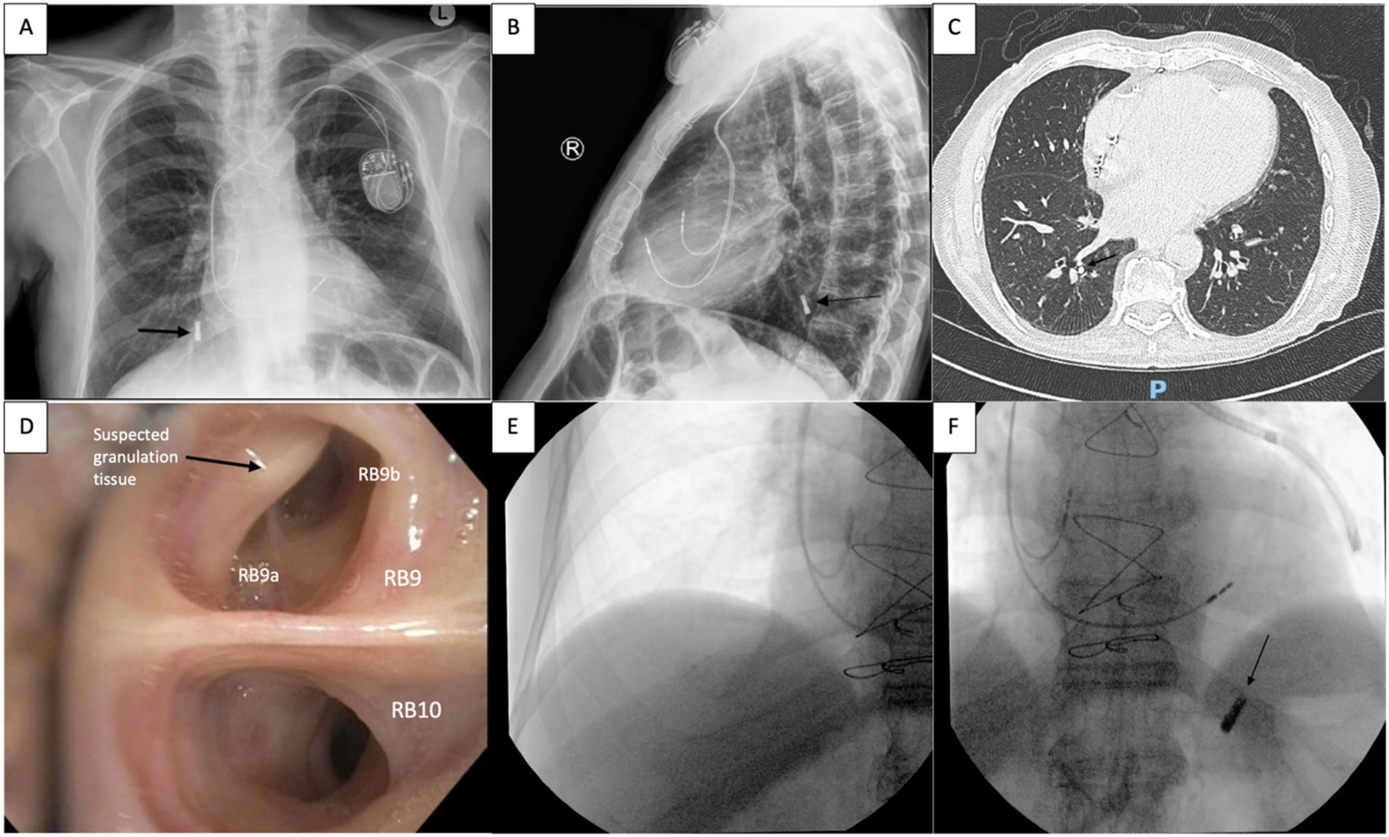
Respiratory phase: (A, B) Chest X‐ray showing metallic foreign body in the right lower lobe → (C) CT Chest confirming foreign body within distal posterior basal segment of right lower lobe → (D) Bronchoscopic view of right lower lobe showing suspected granulation tissue in RB9 and failure to identify foreign body → (E, F) Fluoroscopic migration of foreign body to left hemithorax.
